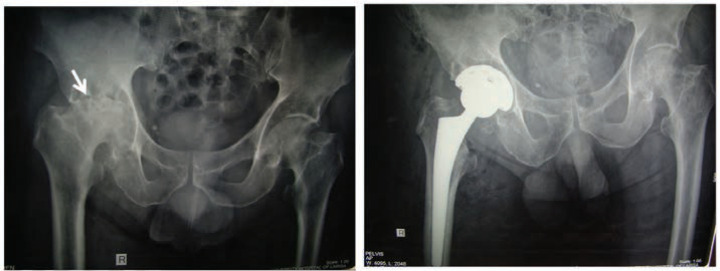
(a) Plain hip X-ray with complete destruction in the hip joint, significant bone degeneration and collapse of the femoral head in the acetabulum (before surgery). (b) hip joint after right total hip arthroplasty.
